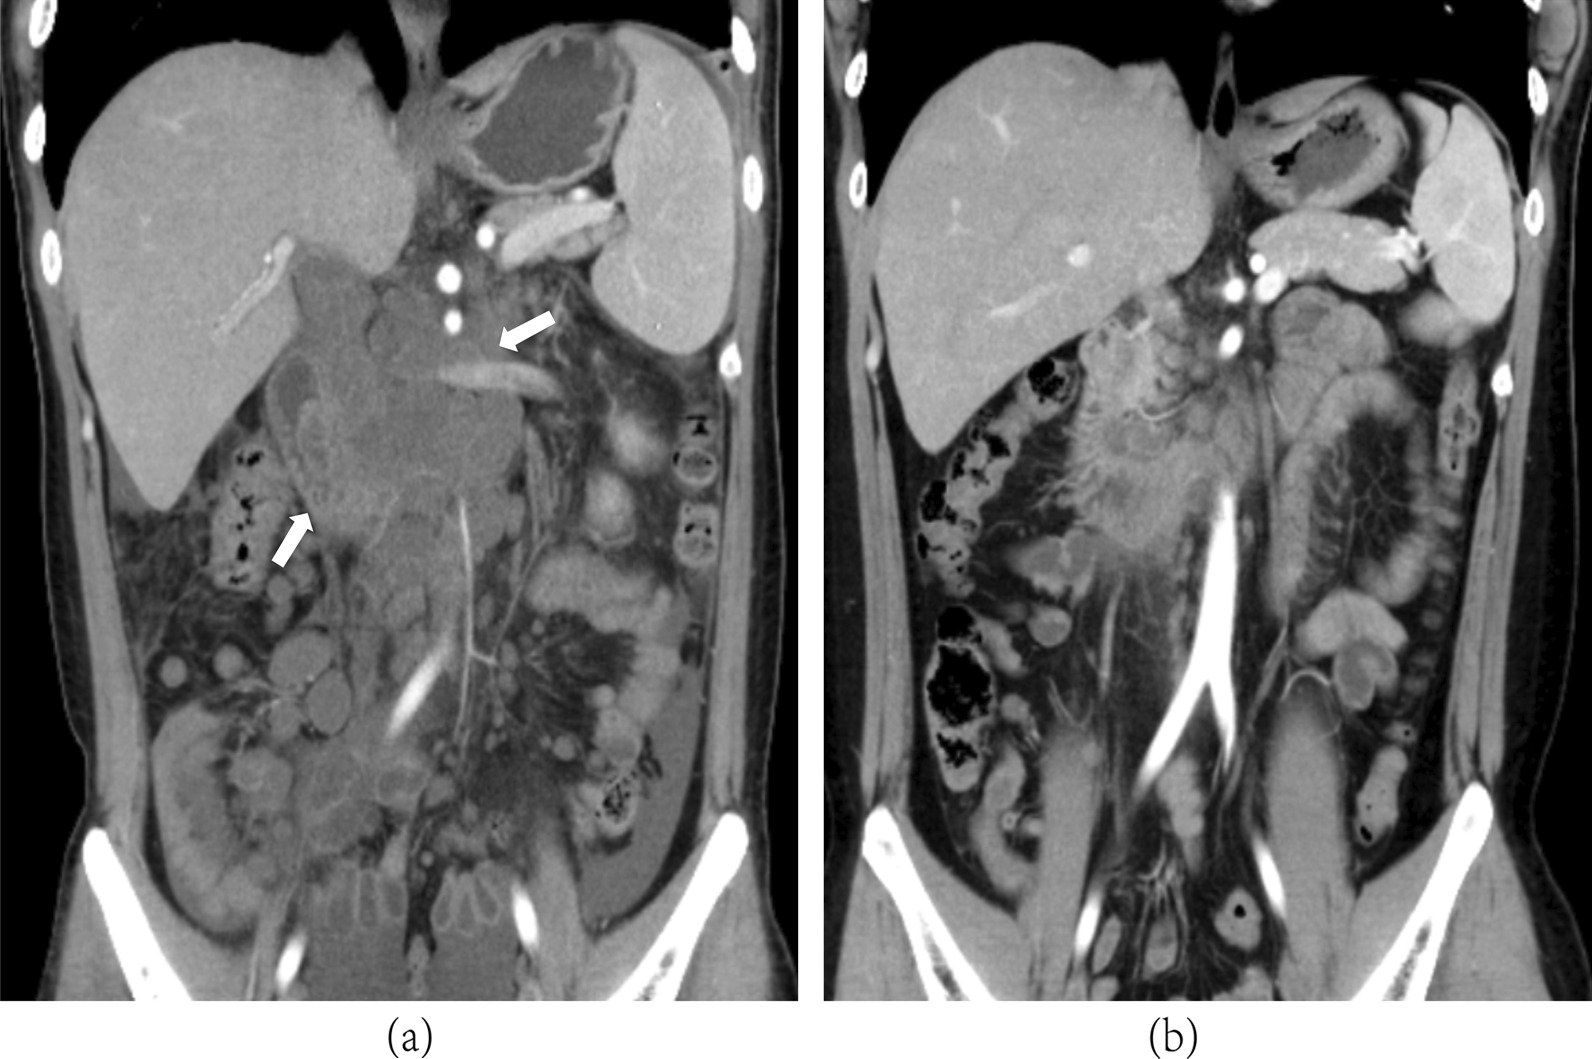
Abdominal CT scan (a) shows a giant mass in the upper abdomen, which demonstrated shrinkage after treatment (b)
